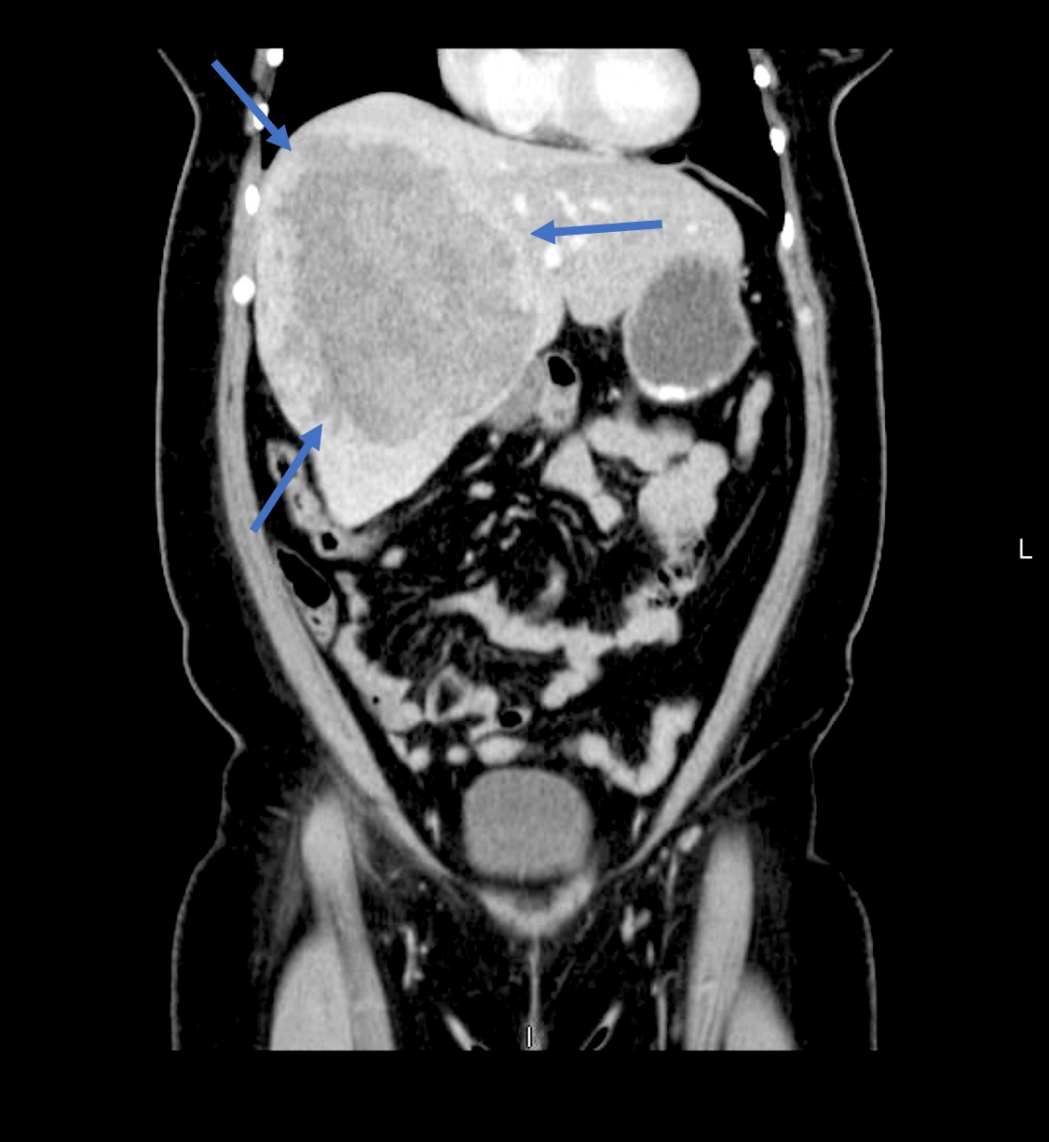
Computed tomography on the day of presentation. Computed tomography demonstrating a 16 cm x 13 cm x 13 cm right liver mass with central stellate scar consistent with focal nodular hyperplasia (FNH).
